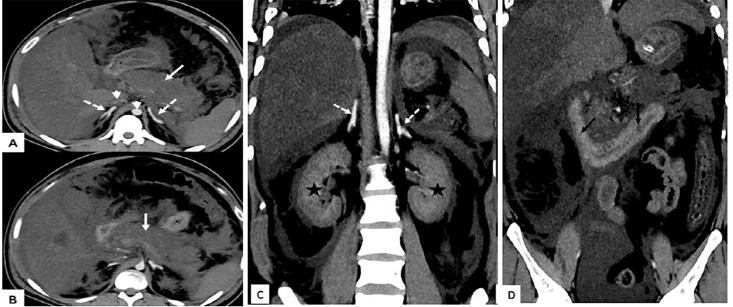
Contrast-enhanced computed tomography abdomen axial (A, B) and coronal (C, D) images showing diffuse pancreatic necrosis (white arrow) with significant peripancreatic inflammation. Intense enhancing bilateral adrenal glands (dashed arrow) with mucosal hyperenhancement of small bowel loops (black arrow) and gross ascites are visible. The short white arrow indicates narrow caliber of abdominal aorta with imperceptible inferior vena cava. Bilateral kidneys (asterisks) are heterogeneously enhancing with perinephric fat stranding likely due to diabetic nephropathy.
